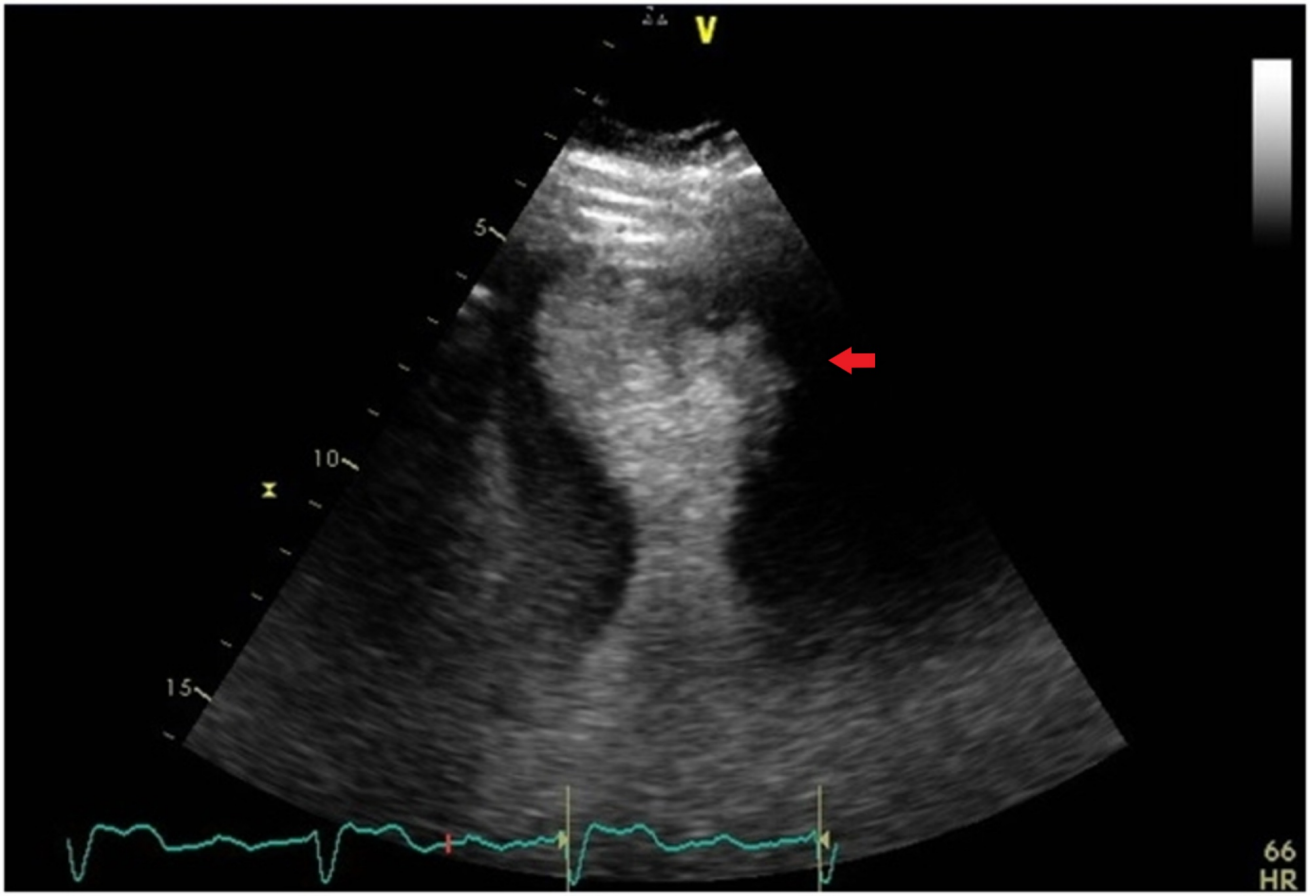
Apical four chamber view showing apical and periapical akinesia and dilation (red arrow) along with preservation of the basilar segment. Moderately increased left ventricular wall thickness was noted too.
